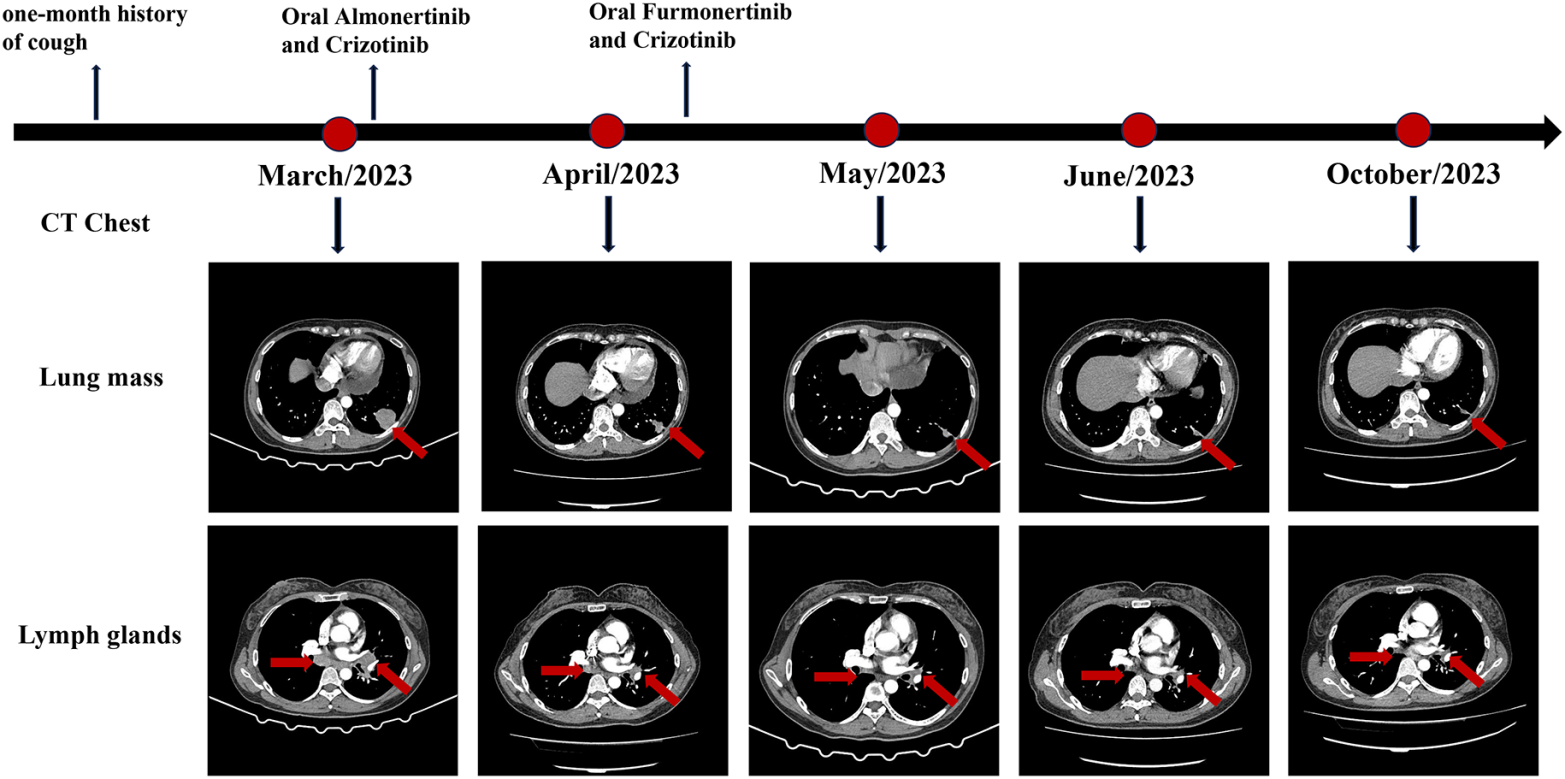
CT scan of the patient throughout the whole course of diagnosis and treatment.
